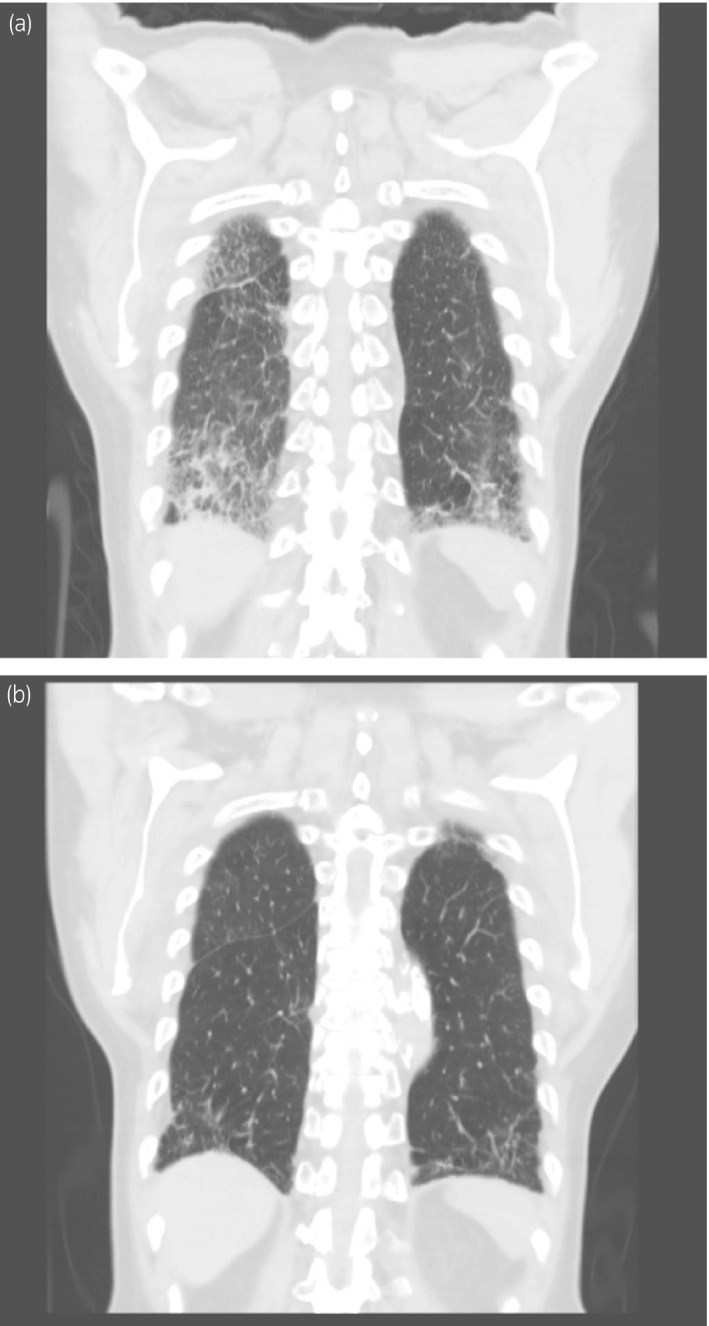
Appearance of chest CT scans of the ILD induced by apalutamide therapy. The apalutamide‐induced ILD (a) was successfully recovered by the steroid therapy (b)
